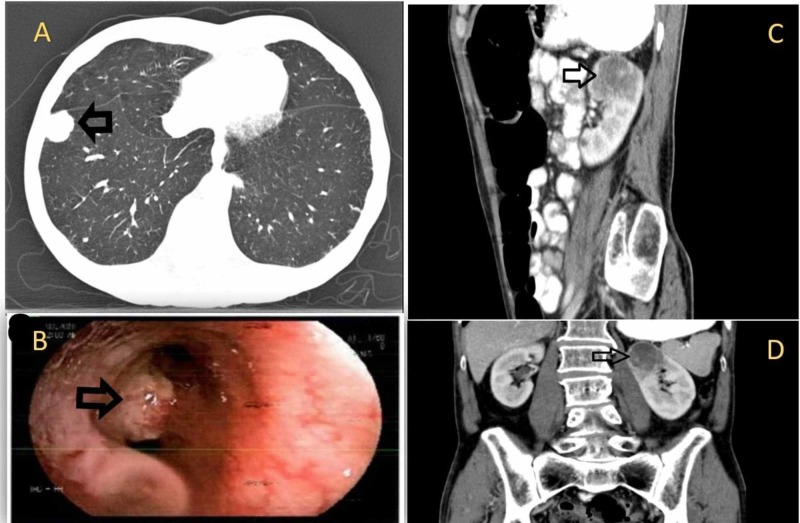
A: CT scan showing the right lower lobe nodule, B: Bronchoscopy image showing the left main bronchus mass, C & D: CT scan showing the isolated left kidney metastasis CT, computed tomography
